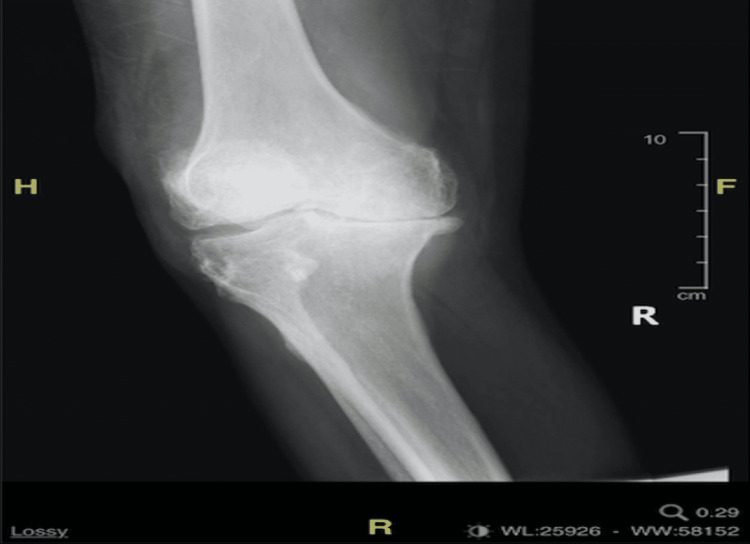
Anteroposterior radiograph of the right knee.
